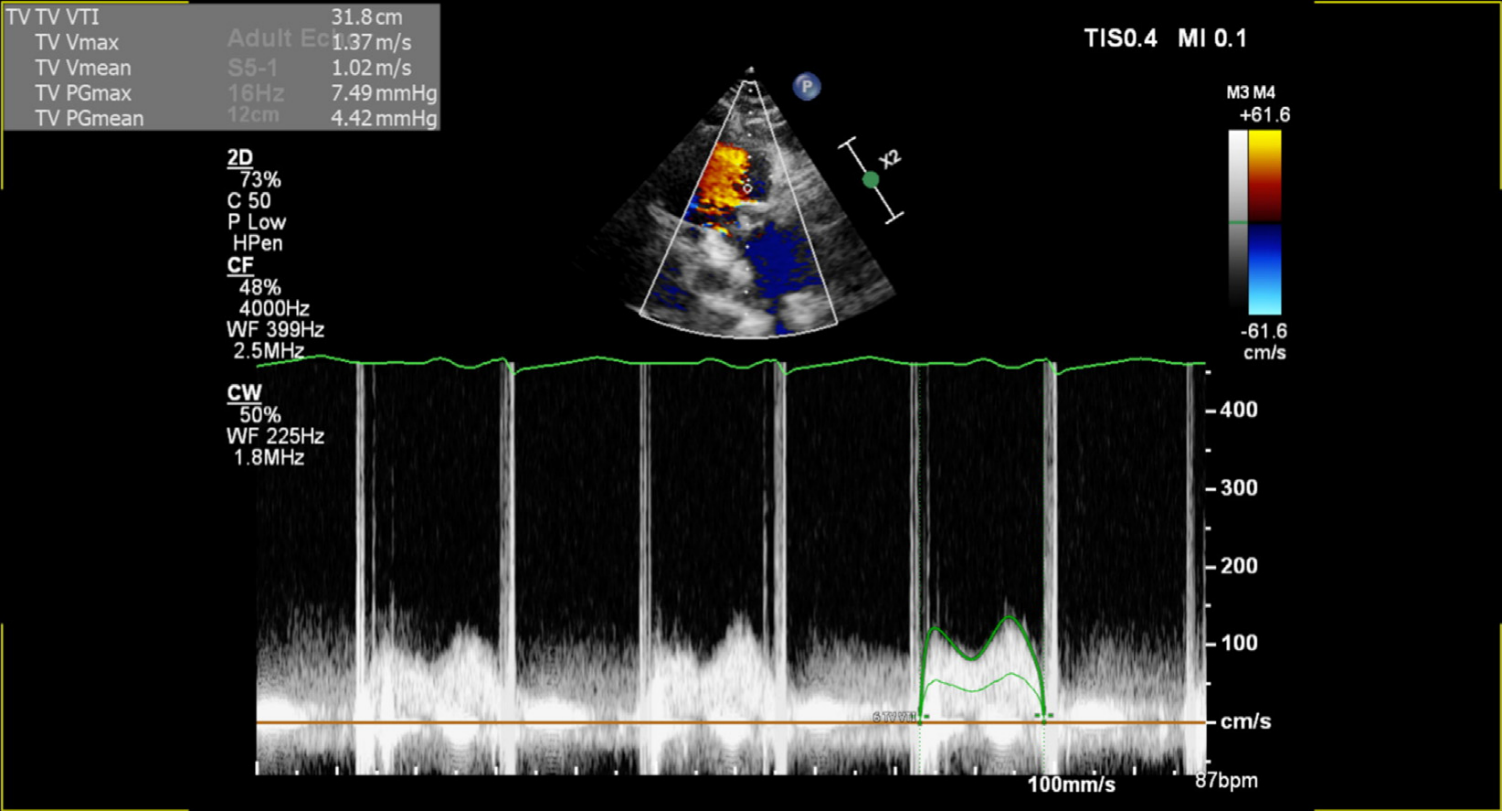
Baseline Transthoracic Echocardiography of Mechanical Tricuspid Valve Replacement With Pulse Wave Doppler in 2023
